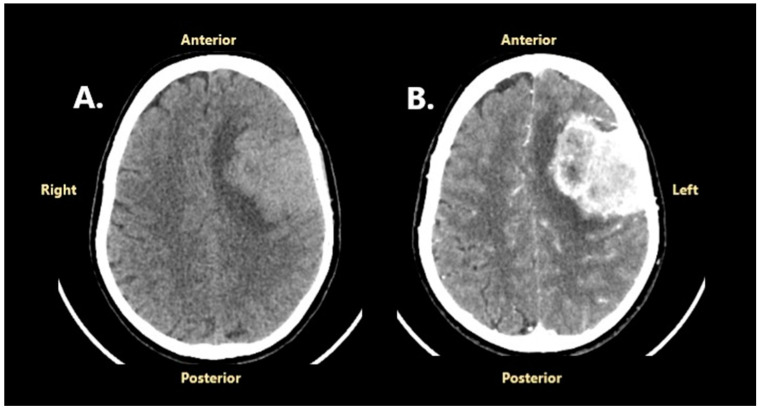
Preoperative cranial CT scan performed in October 2023: (A)—without contrast enhancement; (B)—with contrast enhancement.
